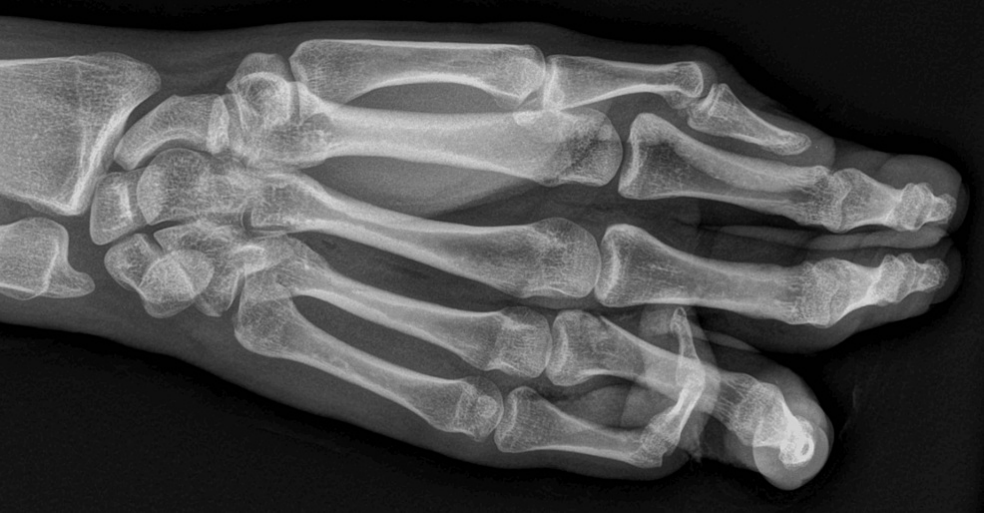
Anterior-posterior radiograph of the hand shows a transverse metaphyseal fracture at the base of the 4th proximal phalanx with dorsal angulation. The fifth digit is uninjured.
